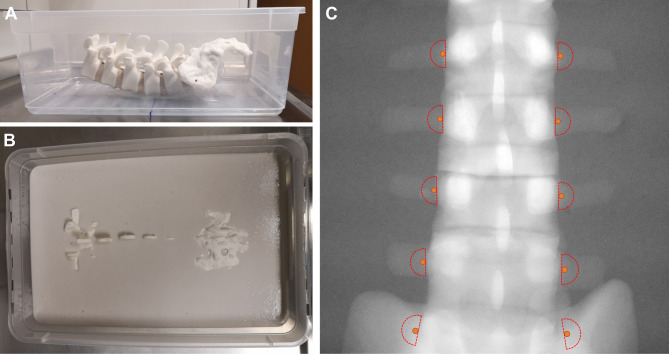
(A) Synthetic lumbar spine model embedded in alginate inside a plastic box. (B) Cast of the box filled with alginate. (C) AP X-ray of the model showing the optimal target points (orange) and the defined tolerance zone (red semicircles), within which the needle tip was accepted during the manual attempt.
